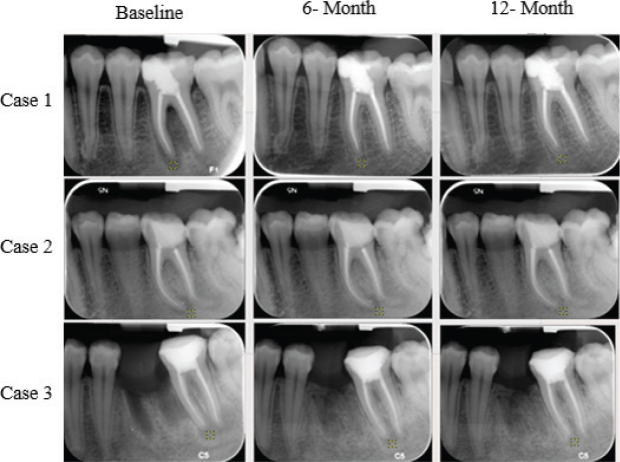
ROI selection for three different cases in periapical radiographs.
